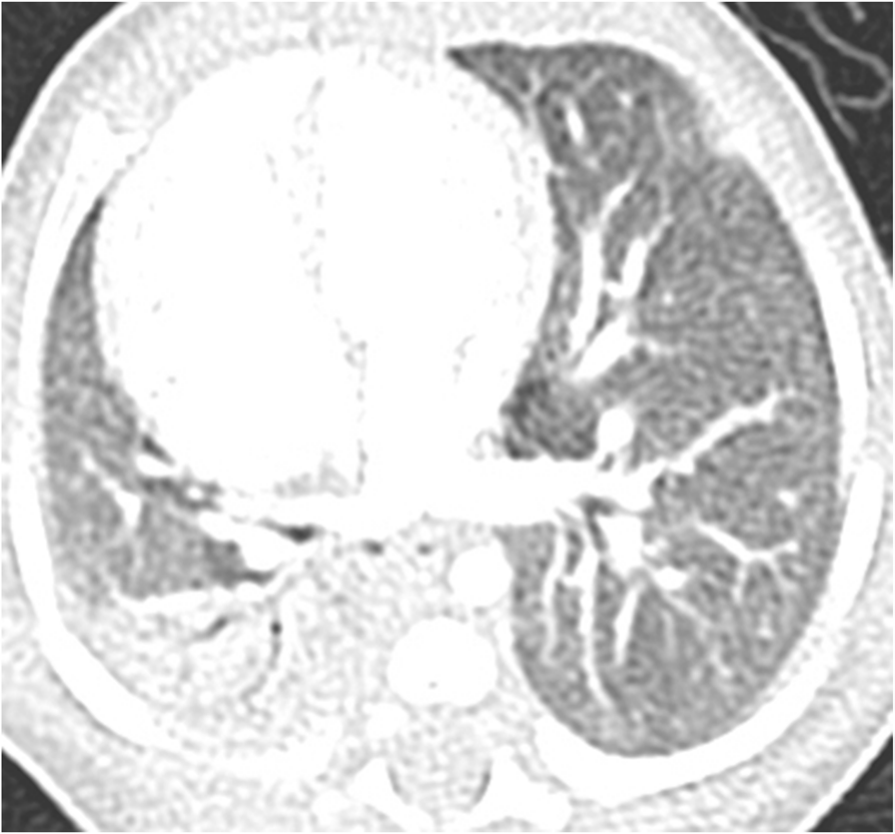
Computed tomography (CT) scan at 1 month of age. It revealed consolidation of the right upper lobe (RUL) and dextrocardia
